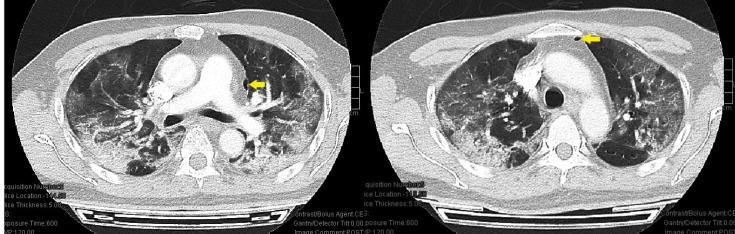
Computed tomography of the thorax showing patchy ground-glass densities
